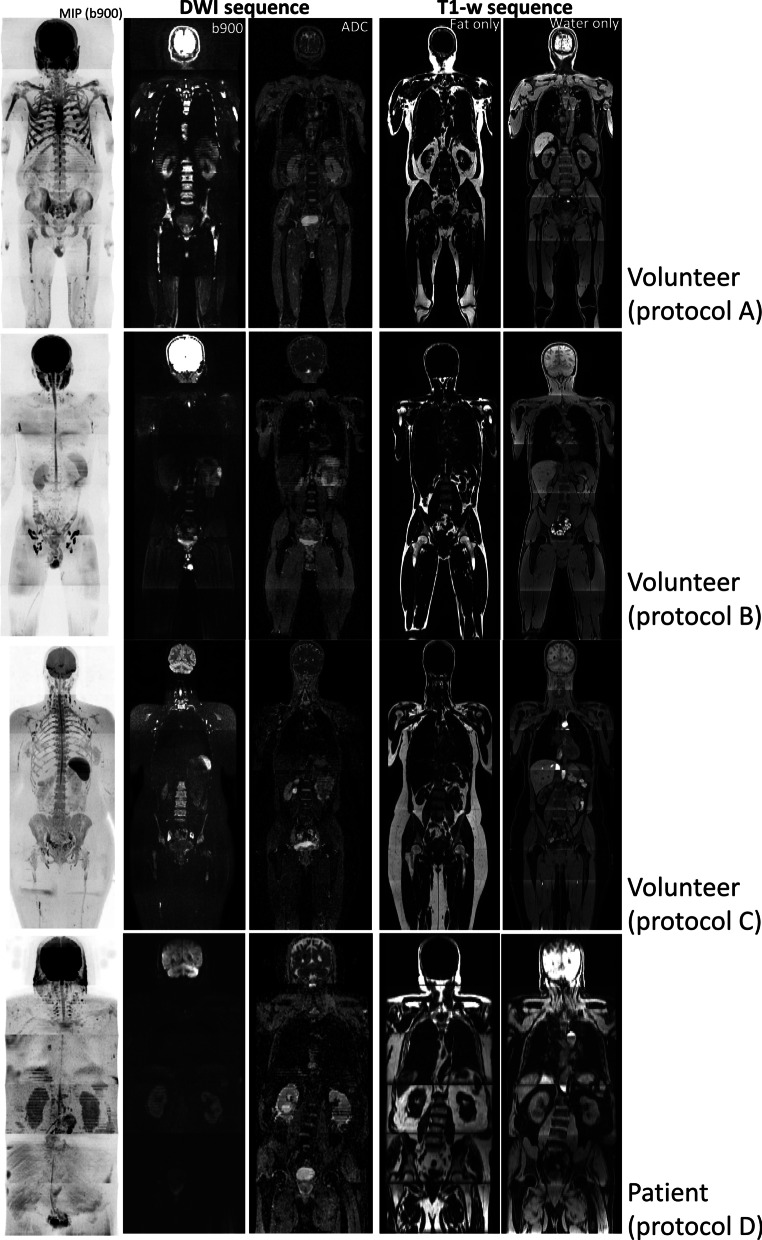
Volunteer/patient images acquired using the four main MR protocols. Coronal reconstructions showing composed MIP, b900 and ADC images (for DWI sequence) and composed fat- and water-only images (for T_1_-w Dixon sequence) for protocol A (Siemens 1.5 T), B (Siemens 3 T), C (Philips 1.5 T) and D (GE 3 T)
